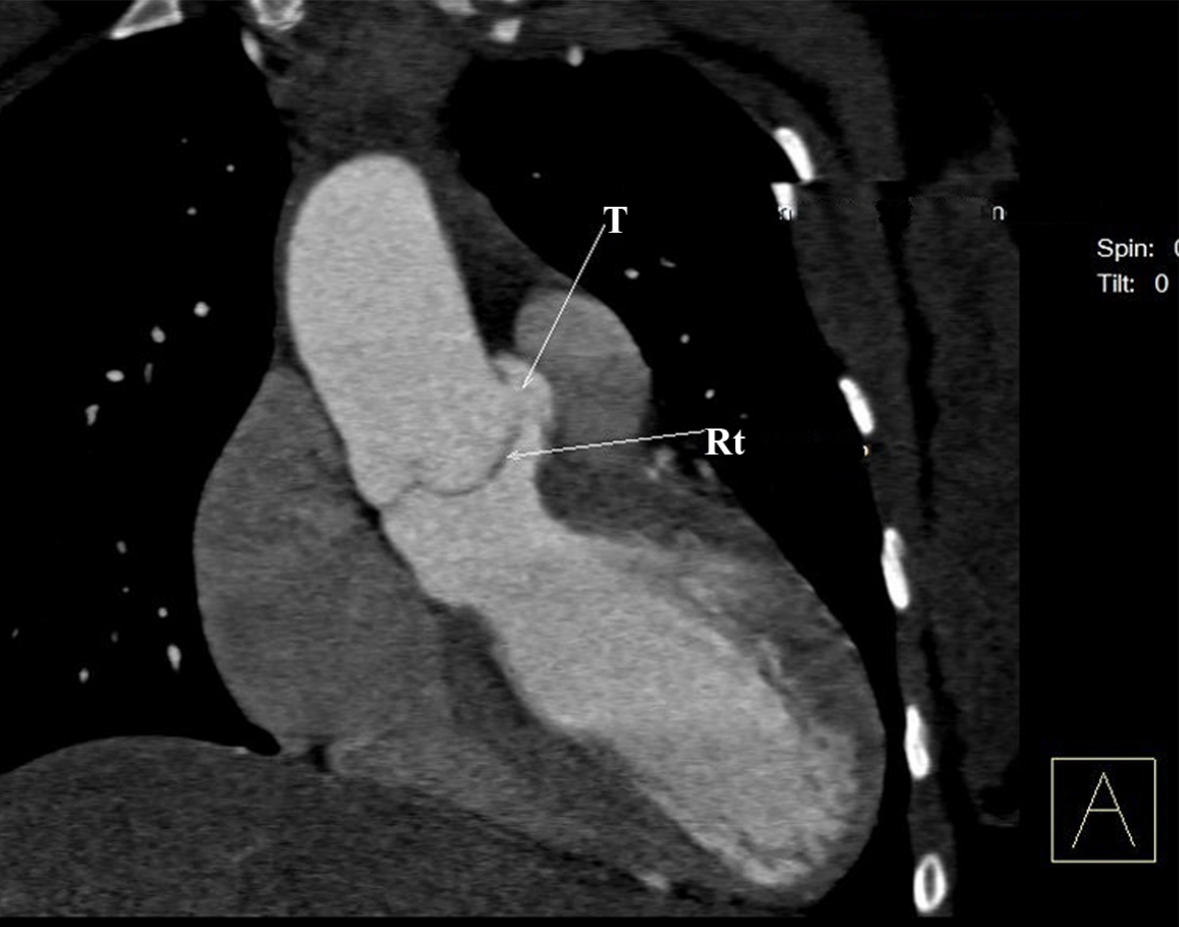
Computed tomography angiography, showing the aortico-left ventricular tunnel (T).
